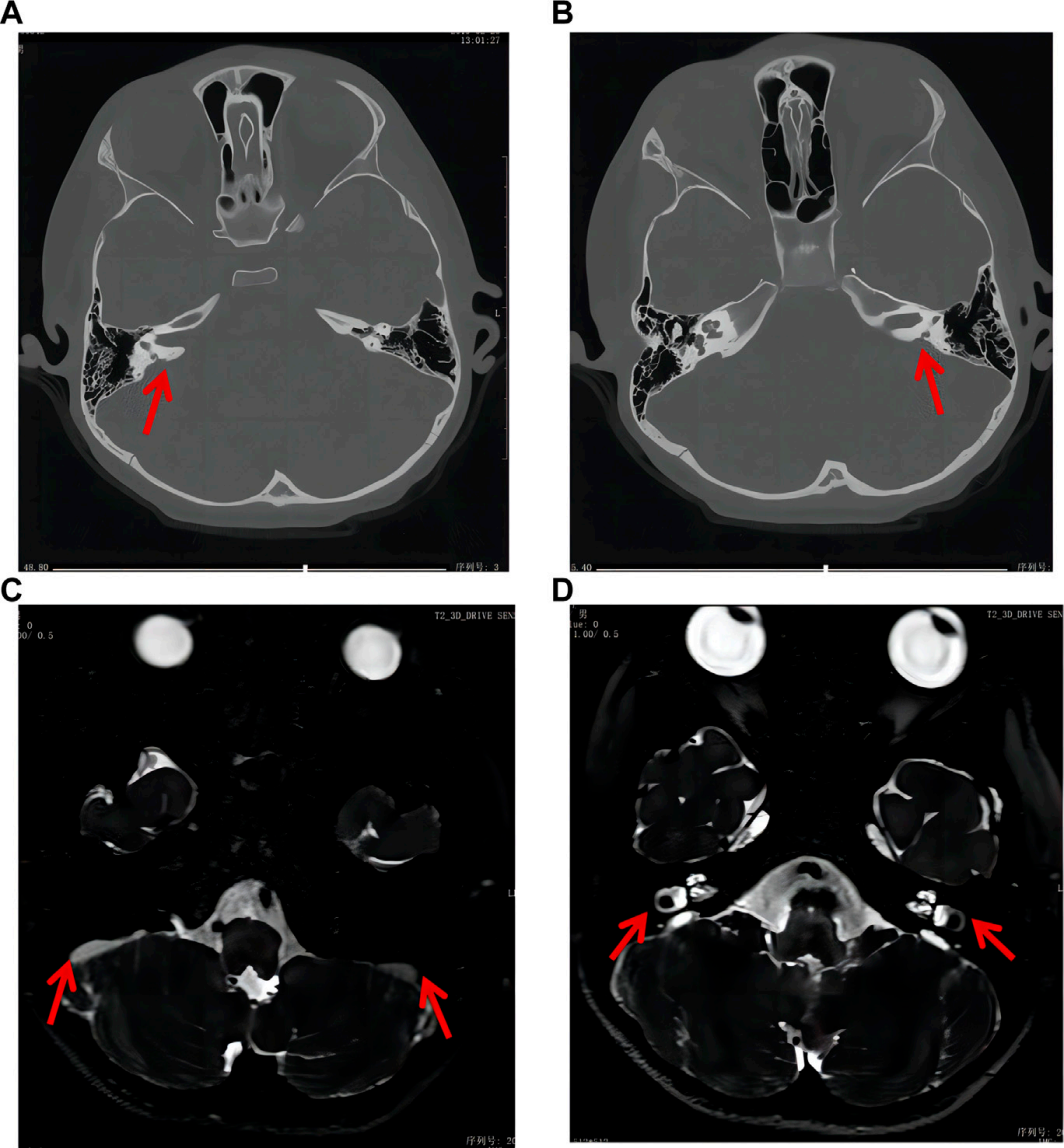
Craniocerebral CT and MRI results of the patient. (A, B) CT results indicated bilateral enlarged vestibular aqueduct (red arrow). (C, D) MRI results indicated bilateral endolymph sac effusion and enlarged vestibular aqueduct (red arrow).
